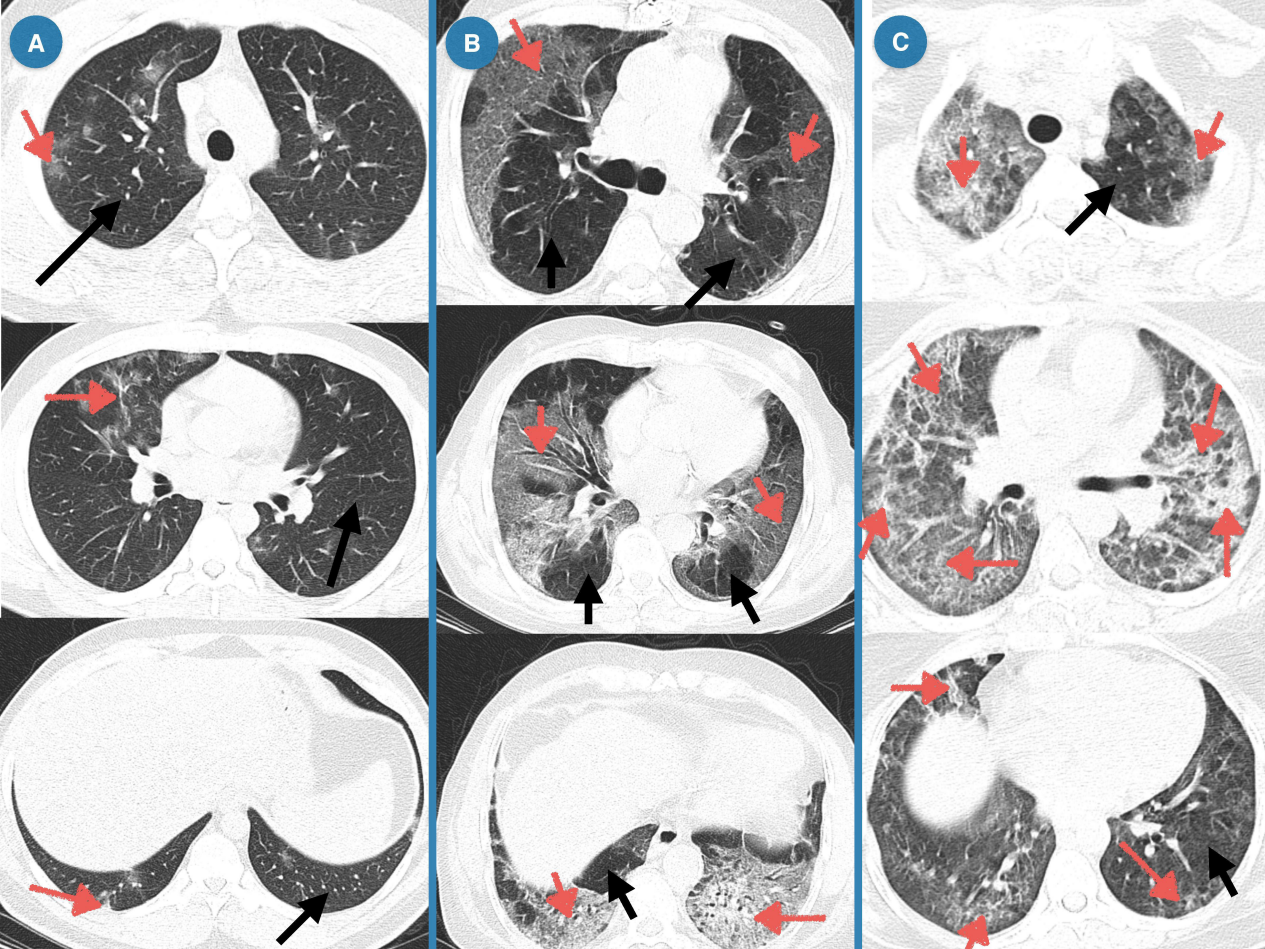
Lung CT of COVID-19 patients representing mild, moderate, and severe respiratory injury. (A) Mild, Multiple peripheral and central ground glass opacities (GGOs) in right upper lobe and left lower lobe; Band atelectasis in left lower lobe; (B) Moderate, Subpleural GGOs near costal and mediastinal pleura of both lungs, Consolidation with air-bronchogram in lower lobes of both lungs, multiple observations of band atelectasis; (C) Severe, Peripheral patchy GGOs, Diffuse and mostly peripheral GGOs, Multiple fibrotic bands.
